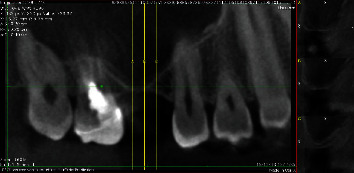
CBCT. Initial situation.
